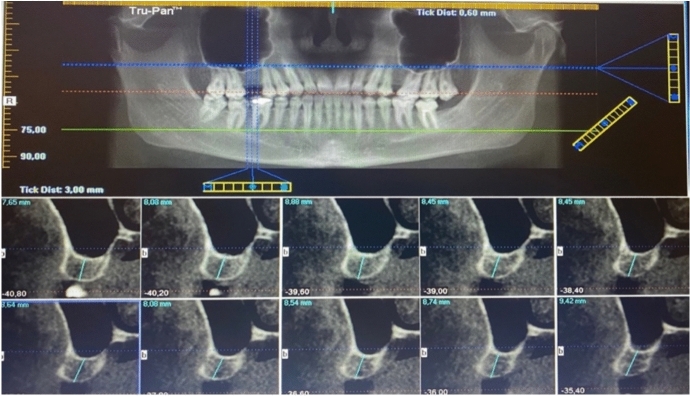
CBCT cross-sectional image demonstrating measurement of RVBH in an edentulous posterior maxilla. RVBH was measured as the vertical distance from the alveolar crest to the floor of the maxillary sinus on cross-sectional slices oriented perpendicular to the alveolar ridge.
